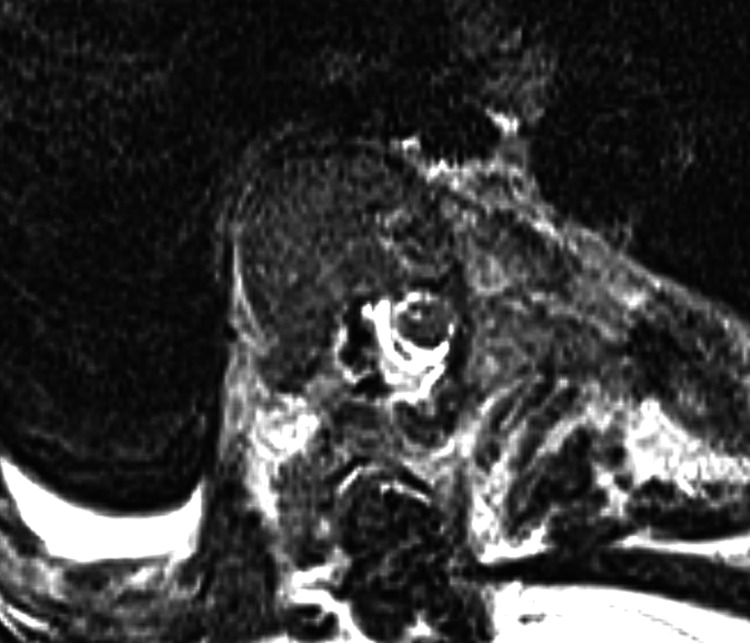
Axial MRI T2 imaging showing a Type 3 spinal cord at the apex.
